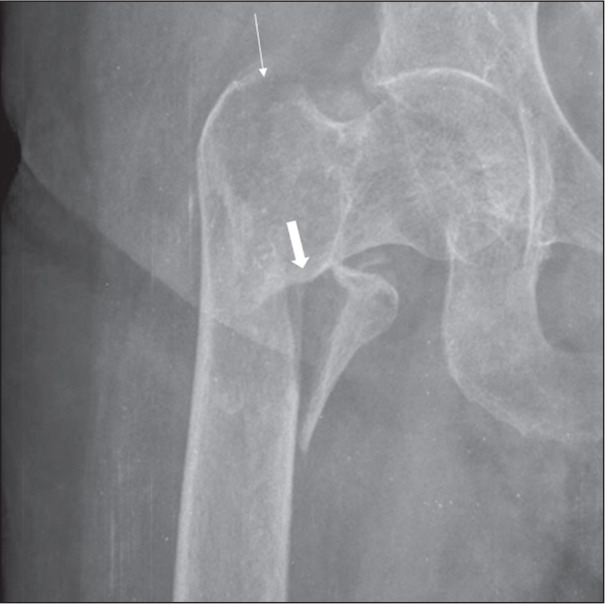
A 63-year-old woman who had fallen out of bed. Anteroposterior X-ray
